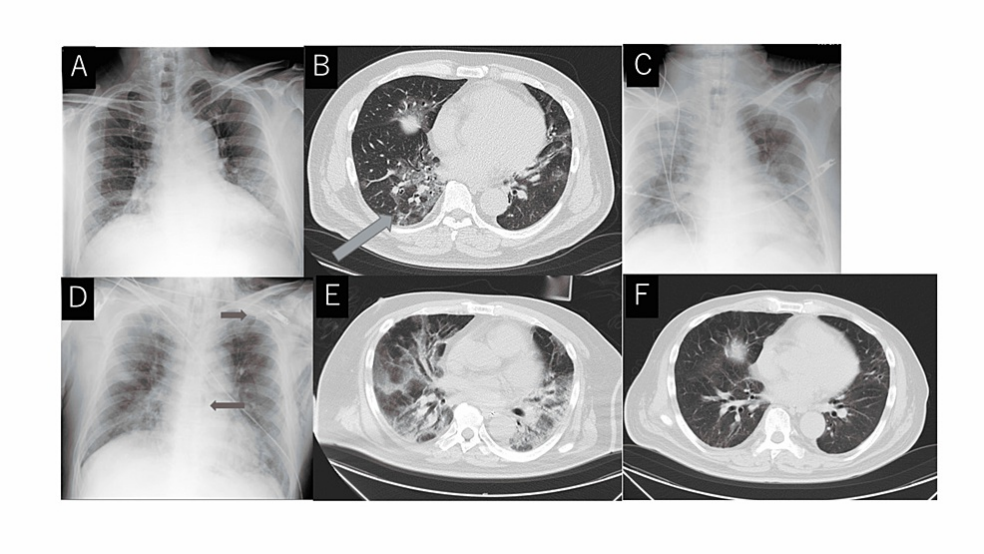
Progressive chest X-ray and chest CT findings A: Chest X-ray on admission, B: Chest CT on admission; a frosted glass shadow was observed in the center of the lower leaves on both sides of the image,  C: Chest X-ray on ICU admission; an infiltration shadow was seen in the bilateral lung fields, D: Chest X-ray after starting extracorporeal membrane oxygenation (ECMO). Mediastinal emphysema and subcutaneous emphysema were observed, E: Chest CT at 34 days of hospitalization (11 days after ECMO withdrawal). Frosted glass shadows were observed throughout the lung field, F: Chest CT at 149 days (prior to hospital discharge); frosted glass shadows remained on both lower lobes but were significantly improved.
